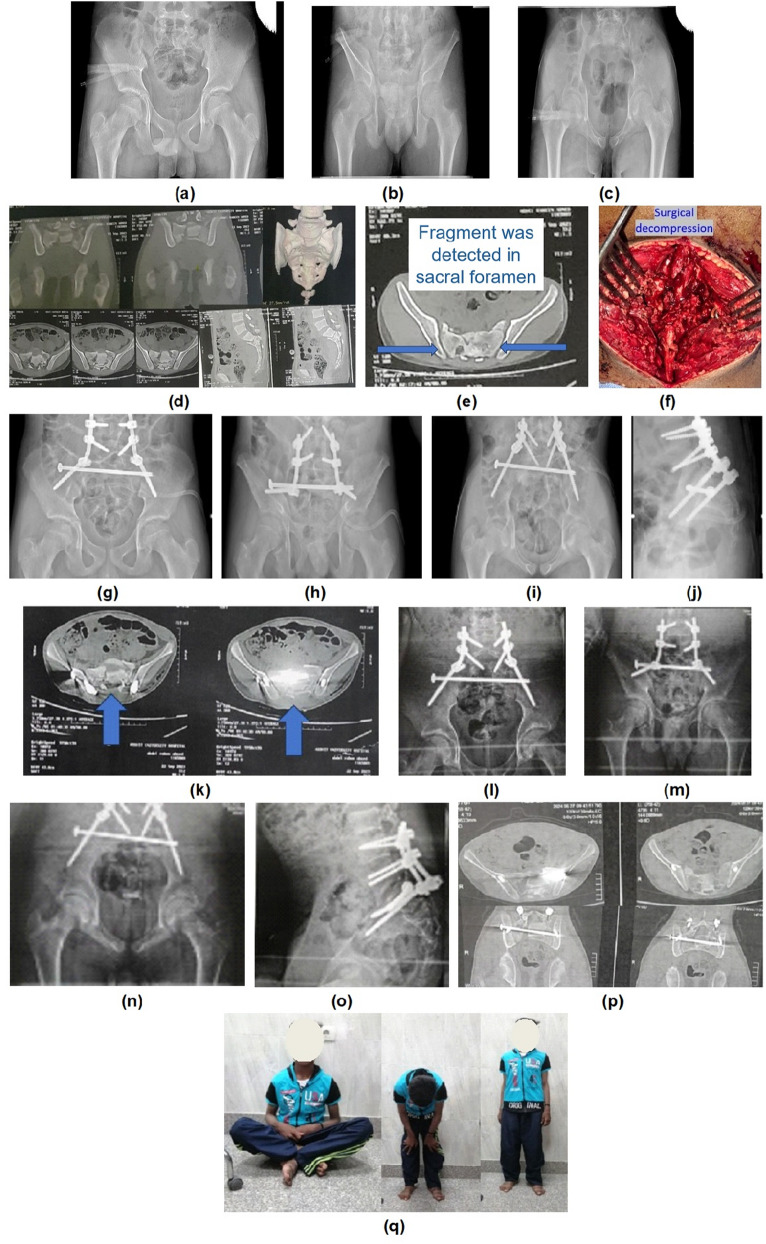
Preoperative (a, b, and c) radiographs of an 18-year patient, (d) Computed tomography showing AO C3 sacral fracture, (e) Preoperative CT showing bony fragment in the sacral foramen, (f) intraoperative photo showing direct decompression through sacral laminectomy, (g, h, i and j) Postoperative radiographs, (k) Postoperative CT showing sacral decompression, (l, m, n and o) Follow up radiographs after 1 year, (p) CT showing fracture union, (q) Clinical and functional outcome after 12 months
